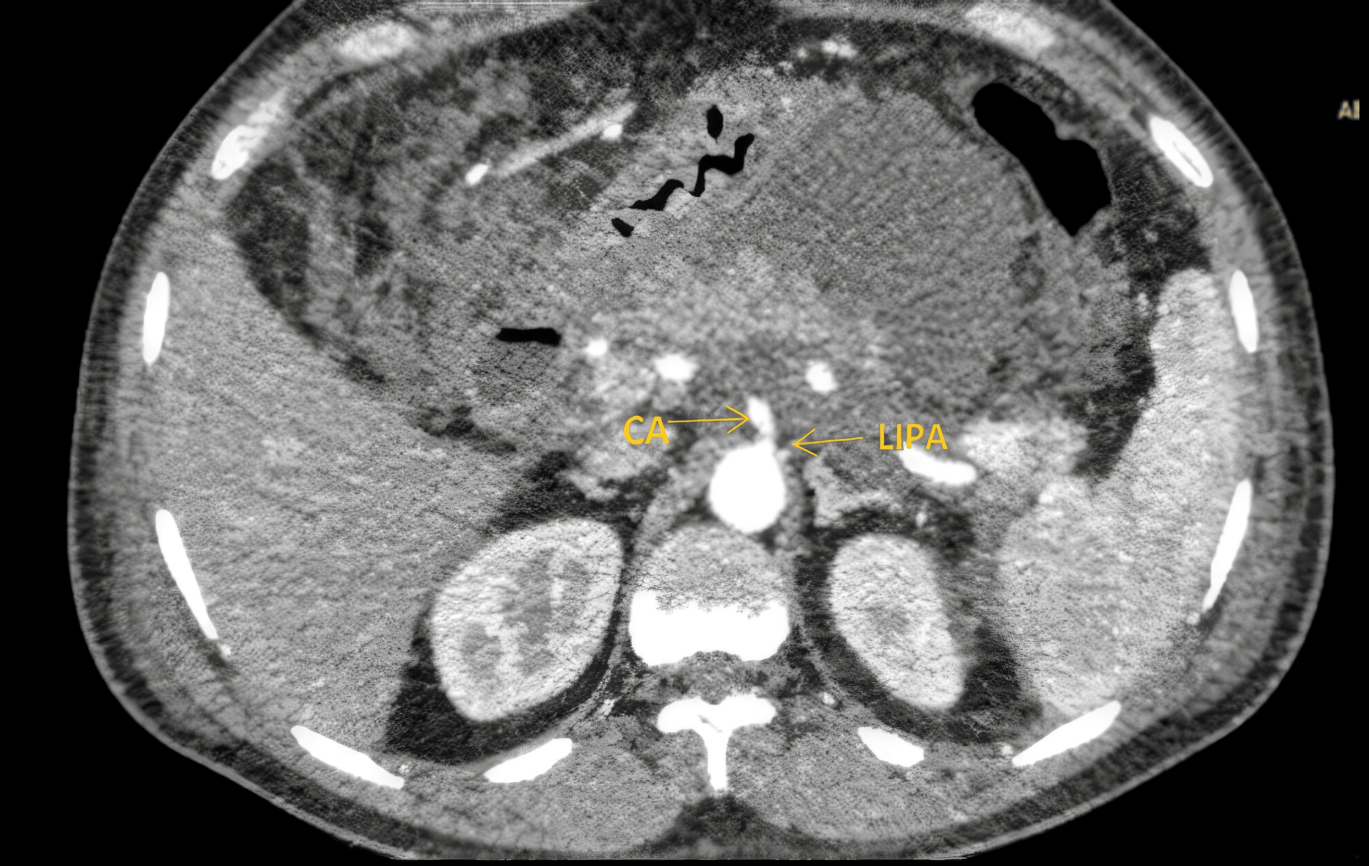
Axial CT image showing the origin of LIPA from the aorta below the level of the CA and above the SMA CA, celiac artery; LIPA, left inferior phrenic artery; SMA, superior mesenteric artery
